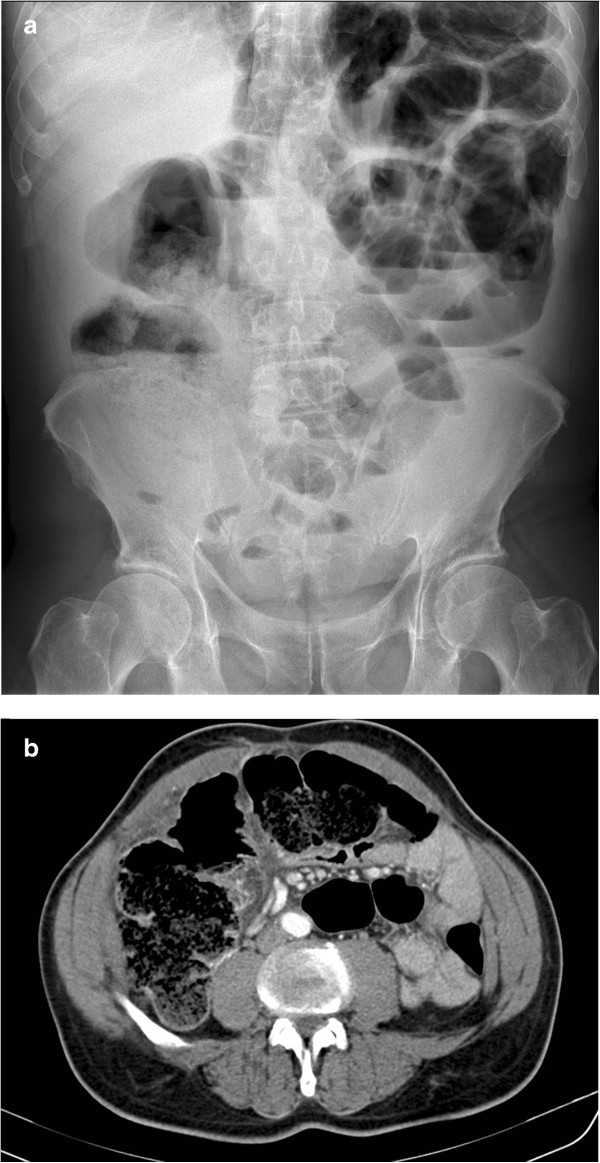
a) X-ray of the abdomen (sagittal plane) showing distension of the small bowel with air fluid levels and no free air. b) Contrast enhanced computed tomography (CT) slide (coronal plane). Extreme distension of the colon. No evidence of free air in the peritoneal cavity.
